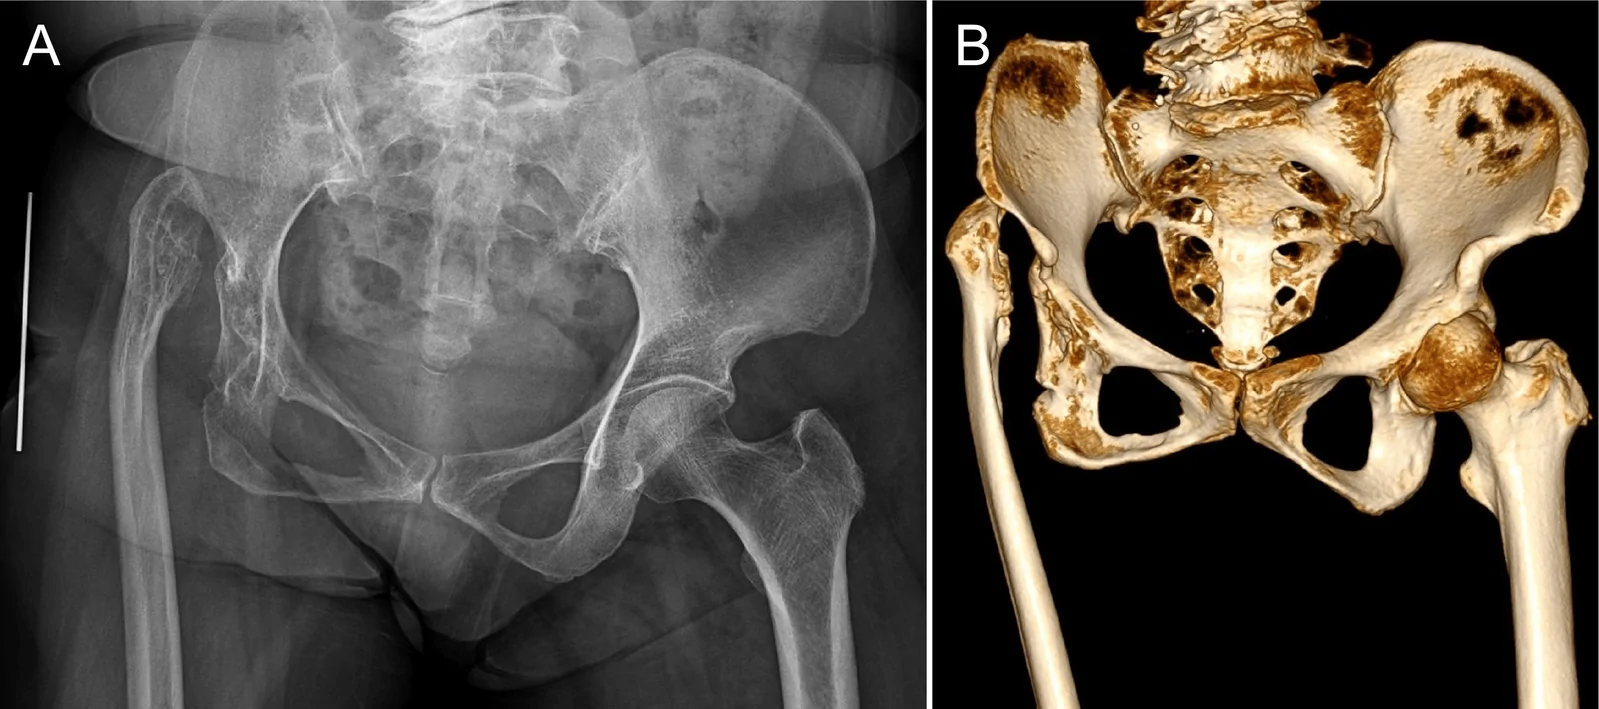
(A) Anteroposterior radiograph of the pelvis, demonstrating a Crowe type IV right hip dysplasia. (B) Three-dimensional computed tomography (3D-CT) reconstruction, demonstrating severe acetabulum bone defect and a narrow femoral canal.
